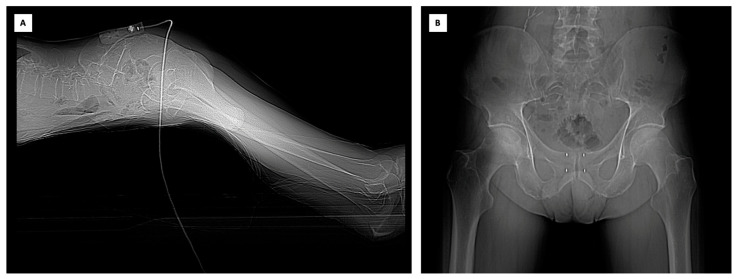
(A) The electromagnetic fiducial marker/field generator applied to the skin of the patient in prone position. (B) The CT confirmed the correct positioning of the markers.
